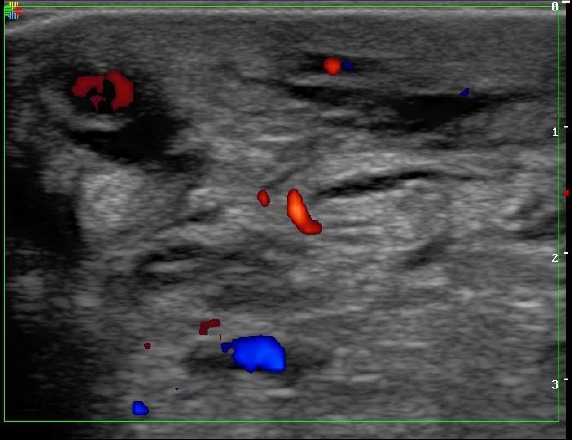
2D Colour Doppler showing thickened soft tissue of the right foot with multiple hypervascular lakes-angiomas and obstructed lymphatic channels*.
